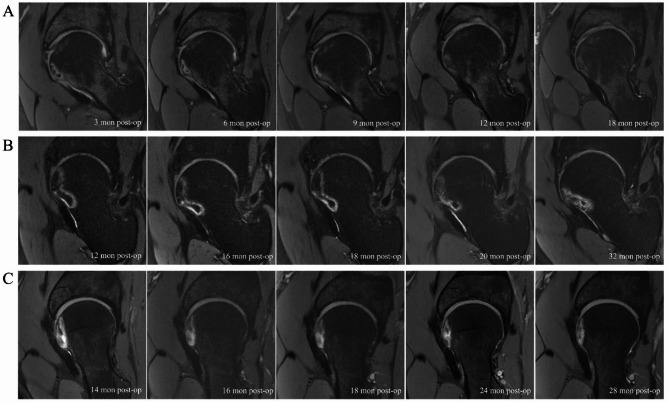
MRI assessment of patients with subsequent follow-ups. Note. Dynamic MRI changes in three patients identified with FHI during MRI follow-up. A-C represents three different patients. FHI, femoral head infarction; MRI, magnetic resonance imaging
